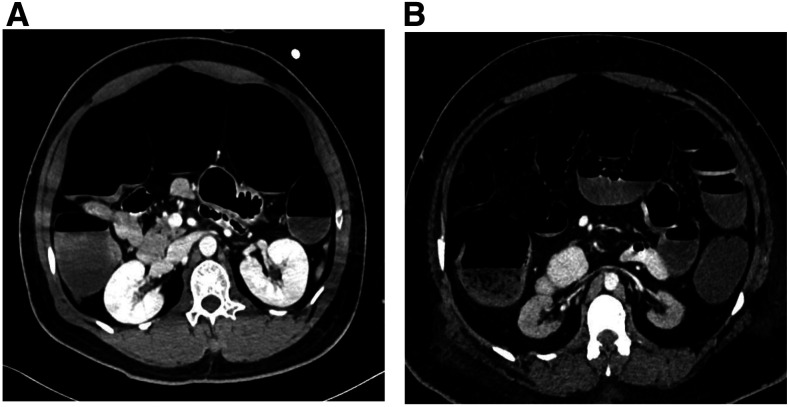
Computed tomography (CT) scan of the abdomen in patients diagnosed with COVID-19 pneumonia. (A) Contrast-enhanced CT scan, an axial plane of the abdomen of the first case showing large bowel dilatation with no stricture or obstruction, and patent mesenteric vessels. (B) Contrast-enhanced CT scan, an axial plane of the abdomen of the second case showing diffusely distended small and large bowel with air–fluid levels.
